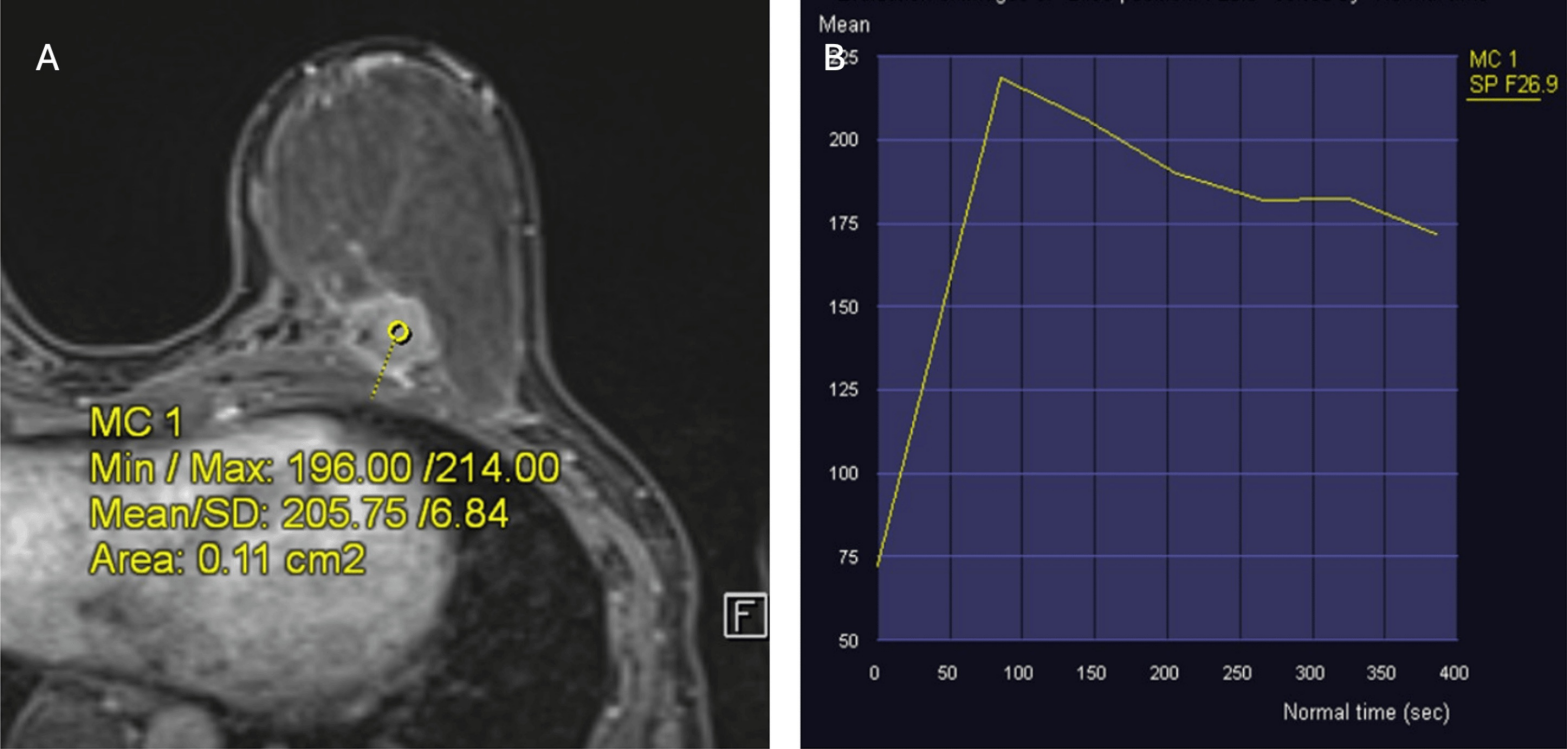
Dynamic contrast-enhanced MRI breast showing (A) placement of the ROI within the left breast 7 o'clock lesion rim-enhancing lesion, and (B) Type 3 kinetic curve produced for the corresponding lesion. ROI: Region of interest
